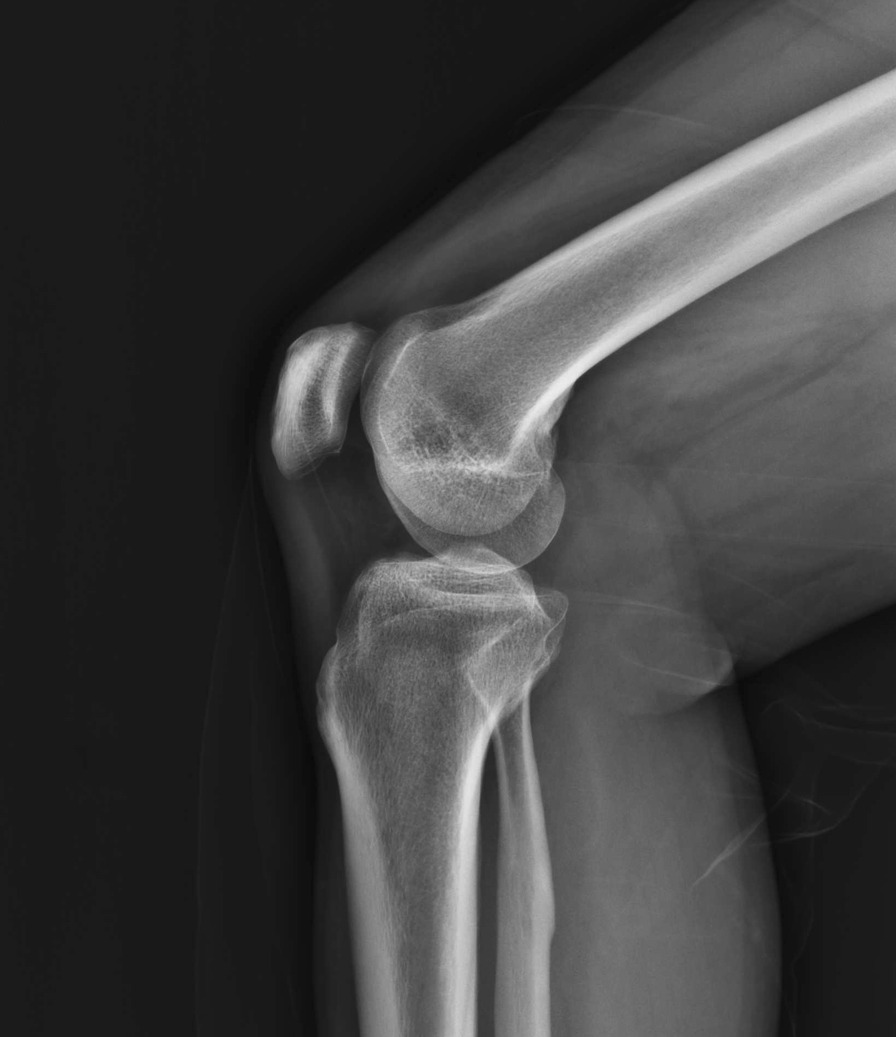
Radiograph of lateral knee joint captured in conventional
